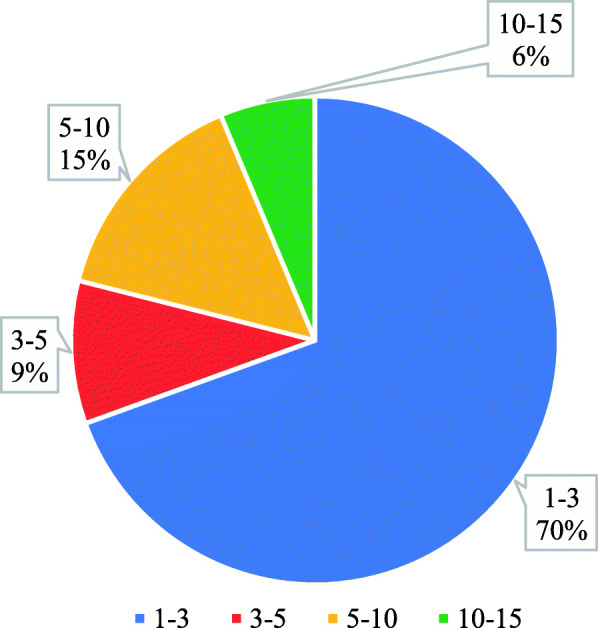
Practical experience of participants in CT scan department (year)
